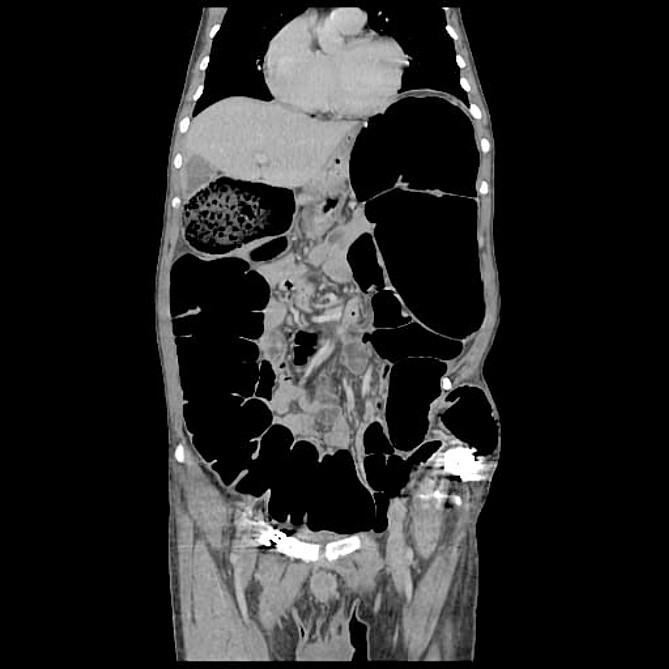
Corona l abdominal CT scan.
